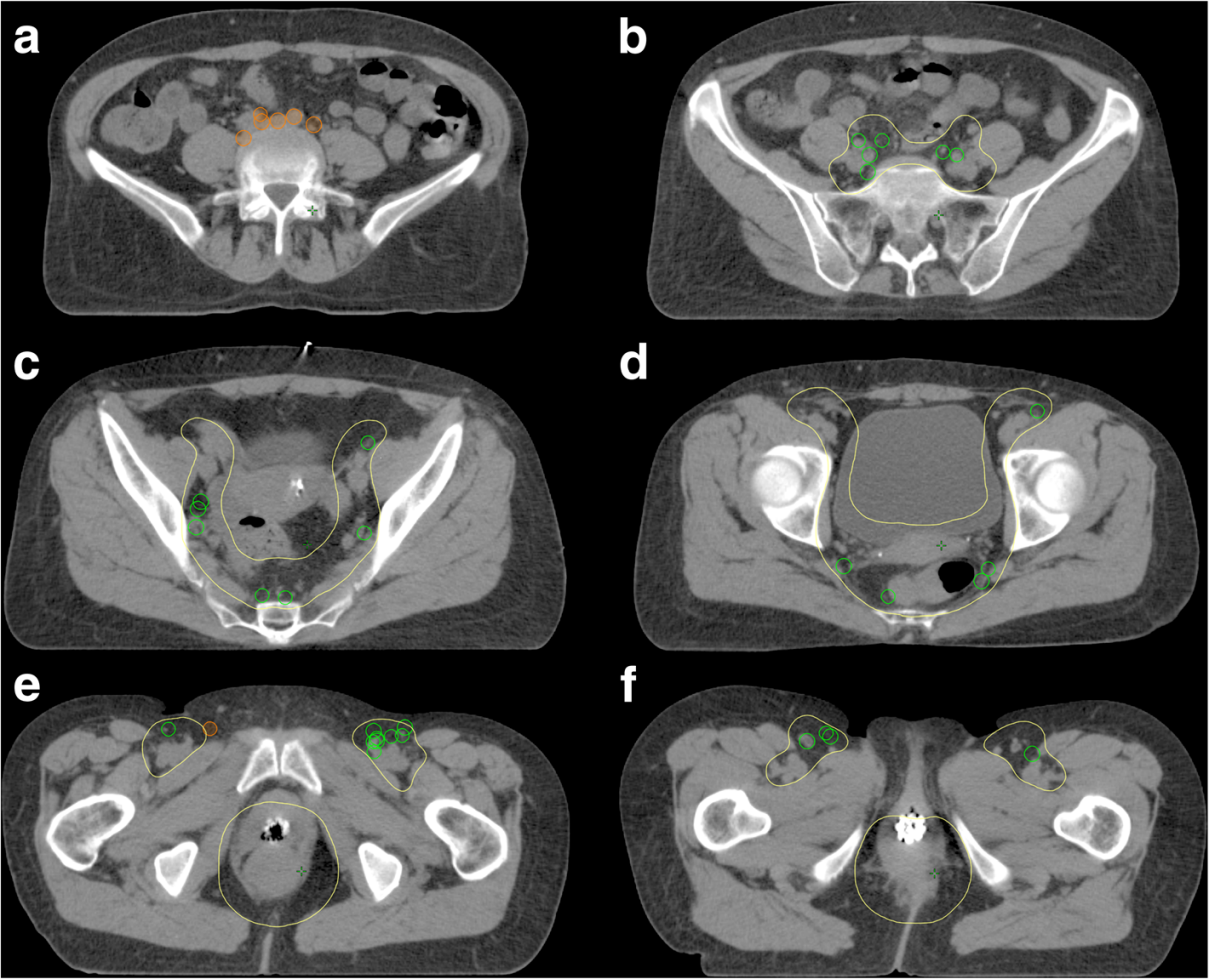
Elective CTV (yellow) as recommended by the RTOG in different CT-slices defined on a standard anal cancer case. Green circles = infield LN. Orange circles = outfield LN.1a: ultimately above the common iliac joint at the height of L5; 1b: first cranial slide at the level of the common iliac joint; 1c: inclusion of external iliac, internal iliac LN and the pre-sacral space above the urinary bladder; 1d: transition of the inguinal and external iliac nodes (lower level of internal obturator artery) with inclusion of the mesorectum, pre-sacral space and the internal iliac LN. Advanced margins (1 cm) into the urinary bladder; 1e: height of the symphysis. Coverage of the inguinal nodes and the anal canal with 2 cm safety margin; 1f: caudal border of the inguinal LN (2 cm below the saphenous/femoral junction) and the primary tumor on primary site
